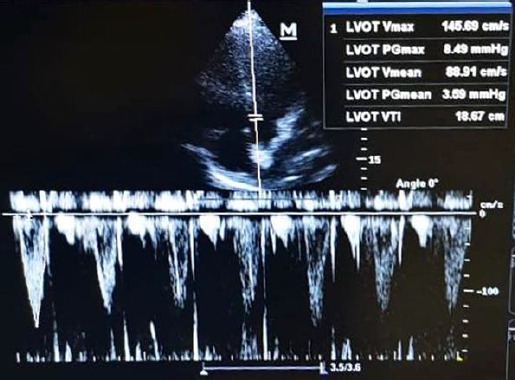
Doppler ultrasound showing left ventricular outflow tract velocity time integral waveform on intensive care unit day 2
